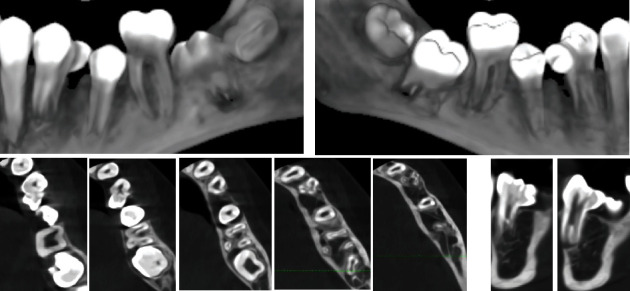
CBCT: 3D vestibular and lingual view and transverse sections.
